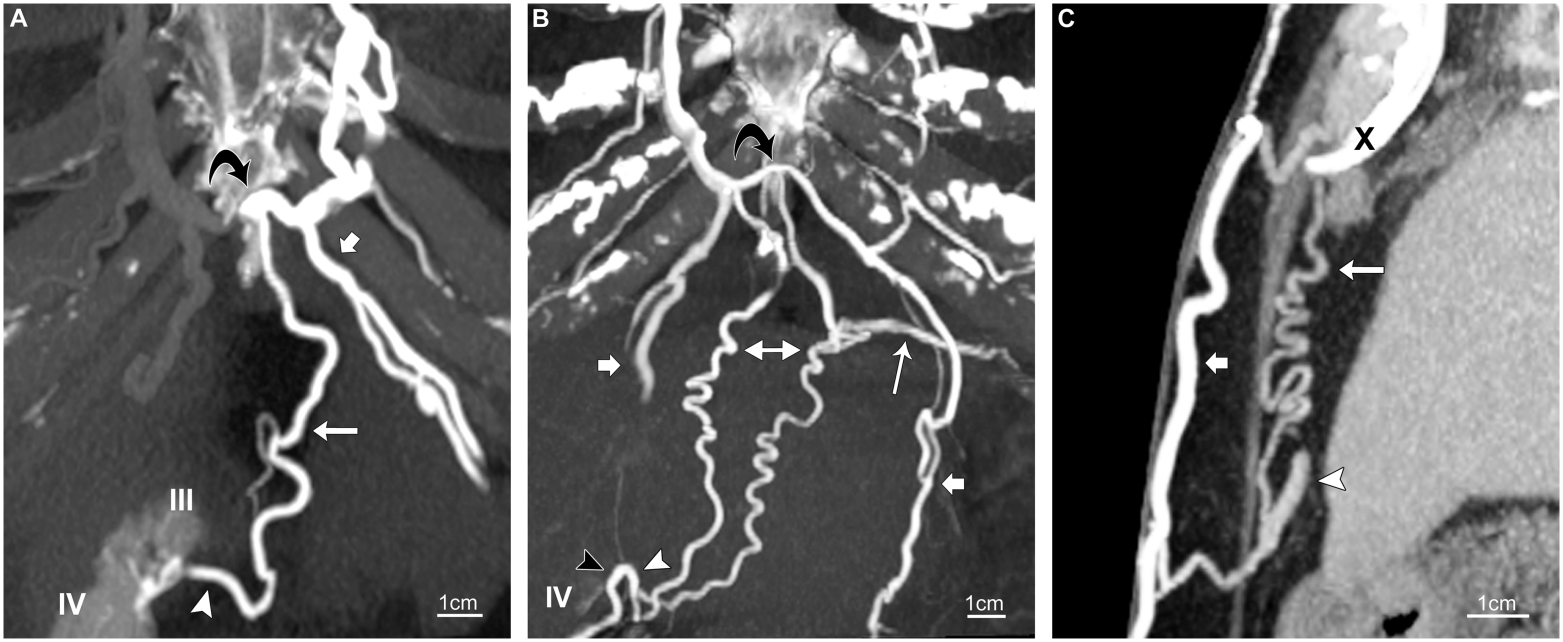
Inferior paraumbilical veins. Routine contrast-enhanced CT scans of chest in three patients with SVC obstruction. (A) 58-year-old woman. Coronal 10-mm maximum-intensity-projection (MIP) shows an inferior paraumbilical vein (arrowhead) terminating at the umbilical notch. It joins subcutaneous veins (not shown), but its only source of collateral flow is an ensiform vein (long arrow) descended from a transverse vessel (curved arrow) between the internal thoracic veins at the xiphoid process. The inferior vein communicates with segments IV and III. The left superior epigastric vein (short arrow) is also shown. (B) 70-year-old woman. Coronal 20-mm MIP shows the confluence of two inferior paraumbilical veins (arrowheads) at the free edge of segment IV. One vessel (black arrowhead) communicates with deep epigastric veins (not shown); the other (white arrowhead) receives two tributaries continuous with ensiform vessels (right-left arrow). The ensiform veins are opacified by the right internal thoracic vein via a transverse branch (curved arrow) across the xiphoid process, and also communicate with left diaphragmatic veins (long arrow). Short arrows point to superior epigastric veins. (C) 76-year-old woman. Sagittal 10-mm MIP shows an inferior paraumbilical vein (arrowhead) destined for segment III. It communicates with a collateral subcutaneous vein (short arrow) across the linea alba and with an ensiform vessel (long arrow). The subcutaneous and ensiform veins both join a transverse vein (not shown) bridging the internal thoracic veins at the xiphoid process (X).
